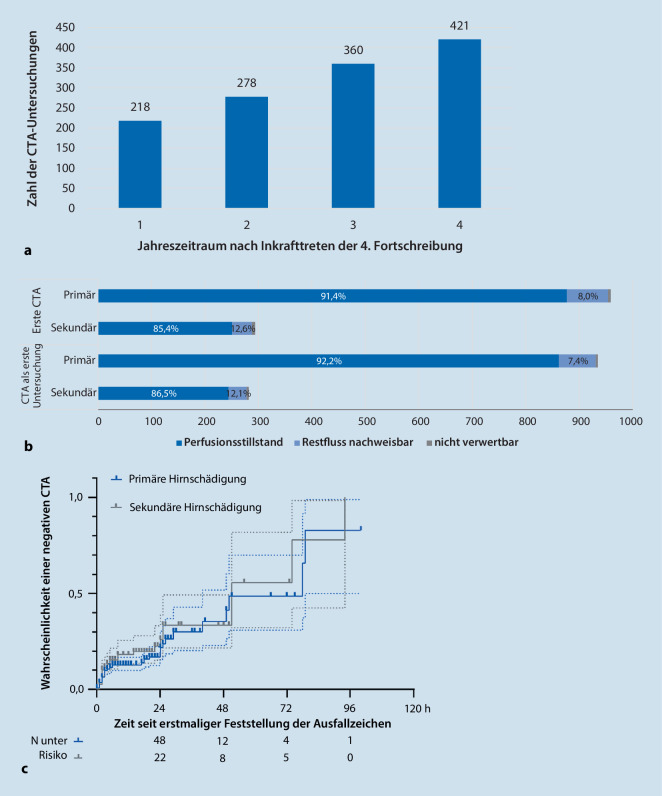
Bei 1217 Patienten erfolgte die CTA als erste ZD. Restperfusion zeigten 103 Patienten (8,5 %). Hiervon erhielten 17 später erneut eine CTA. Positiv waren 7 dieser CTA, während 10 weiterhin Perfusion zeigten. Tendenziell häufiger wurden in dieser Situation positive Befunde mittels EEG (22 von 27) oder Szintigraphie (3 von 3) erhoben, dagegen nur bei 4 von 8 transkraniellen Doppler- bzw. Duplexsonographien (TCD).
